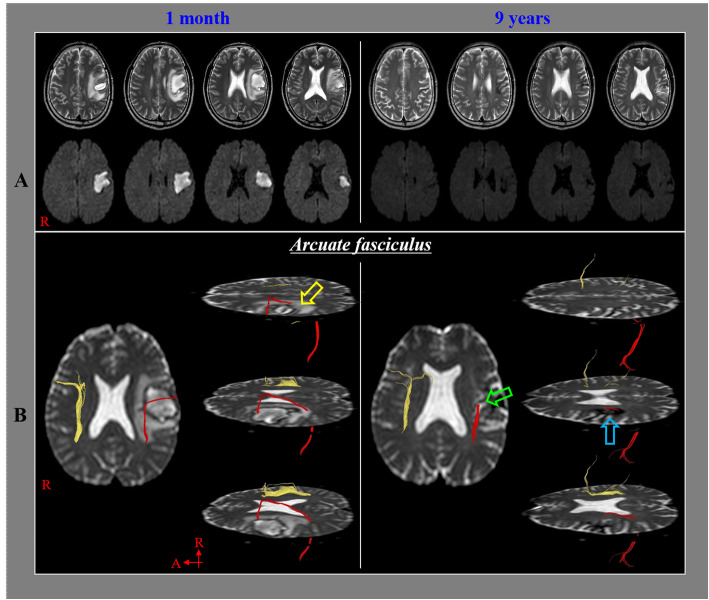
a. T2-weighted and diffusion-weighted brain magnetic resonance images show hematoma presence in the left subcortical area at one month after stroke onset and hemosiderin deposits in the left subcortical area at nine years after onset. b. The integrity of the left arcuate fasciculus was preserved through the hematoma (yellow arrow) on 1-month post-onset diffusion tensor tractography. However, the middle portion of the left arcuate fasciculus showed discontinuation (green arrow) in the middle of an area of hemosiderin deposits (sky-blue arrow) on 9-year post-onset diffusion tensor tractography.
